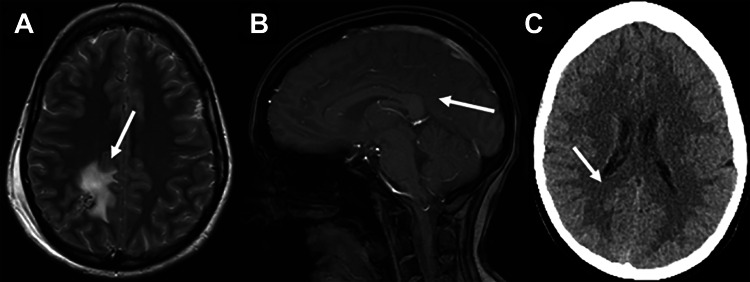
Postoperative images taken at 24 hours, three months, and nine months after surgery, respectively. A: Axial T2-weighted magnetic resonance (MR) imaging of the brain taken 24 hours postoperatively. Residual blood products (arrow) are visible. B: Sagittal MR imaging scan of the brain obtained three months postoperatively. Overall, a near-complete resolution of the T2 signal (arrow) that had been seen in the prior images is demonstrated. C: Noncontrast CT of the head obtained nine months postoperatively showing total resolution (arrow) of the lesion effect.
